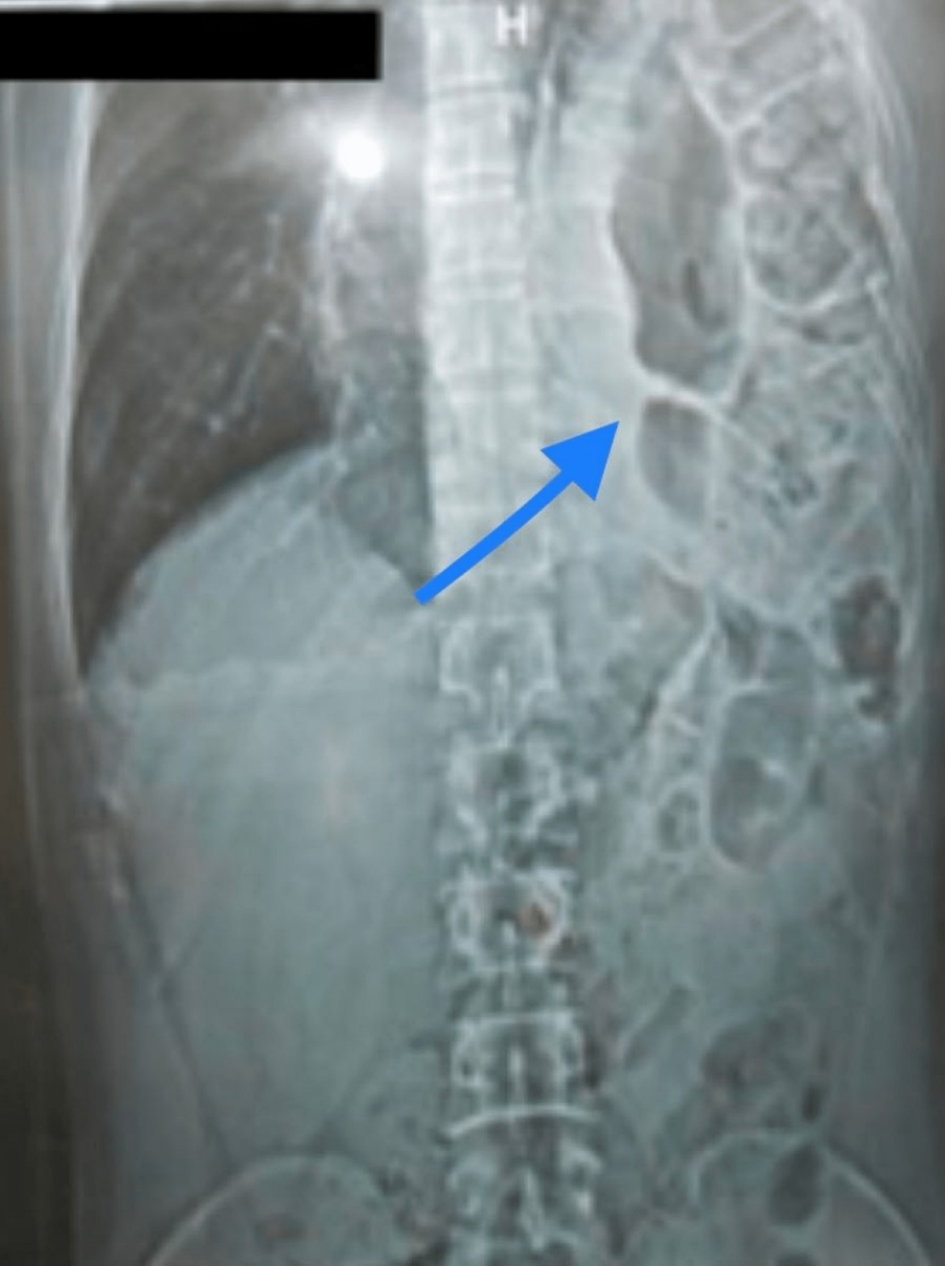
X-ray of the chest and abdomen showing herniation of bowel loops into the left side of the chest with the collapsed left lung.
